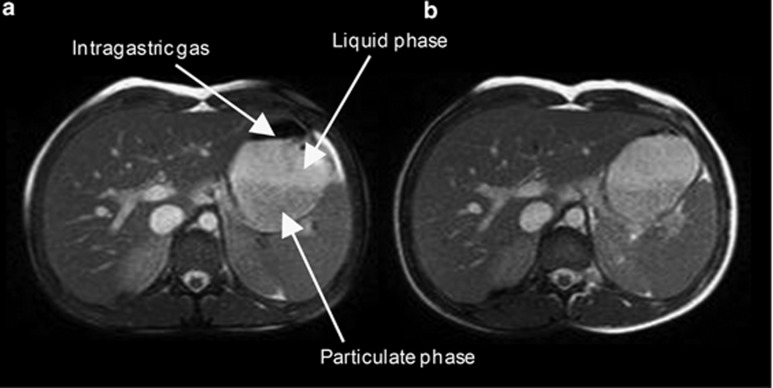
Representative example MRI images of the stomach of a volunteer taken at the first time point after eating (a) the high-carbohydrate and (b) the high-fat meal on two separate occasions. In the images of the stomach the less intense lower portion belongs to the particulate phase of the meal while the brighter upper phase belongs to a fluid layer. The black part on the top of the stomach is a pocket of intra-gastric gas.
